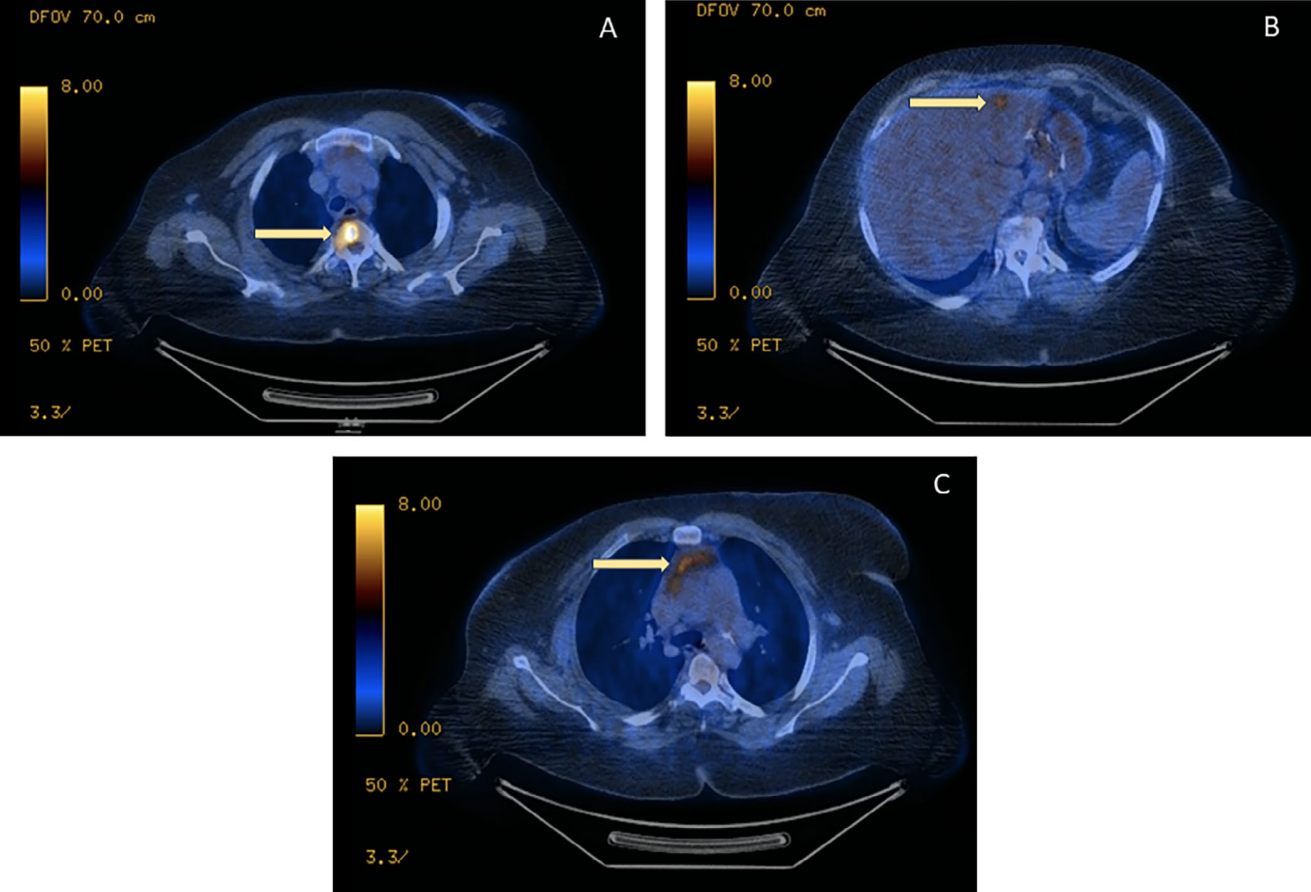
(A‐C). PET‐CT scan demonstrating FDG‐avid lesions at the T2 vertebra (SUV max 10.2) (A), left hepatic lobe (2.7 x 2 x 2.8 cm, SUV max 5.2) (B), and anterior mediastinum (3.6 x 1.5 cm, SUV max 3.1) (C), all highlighted by bold yellow arrows.
